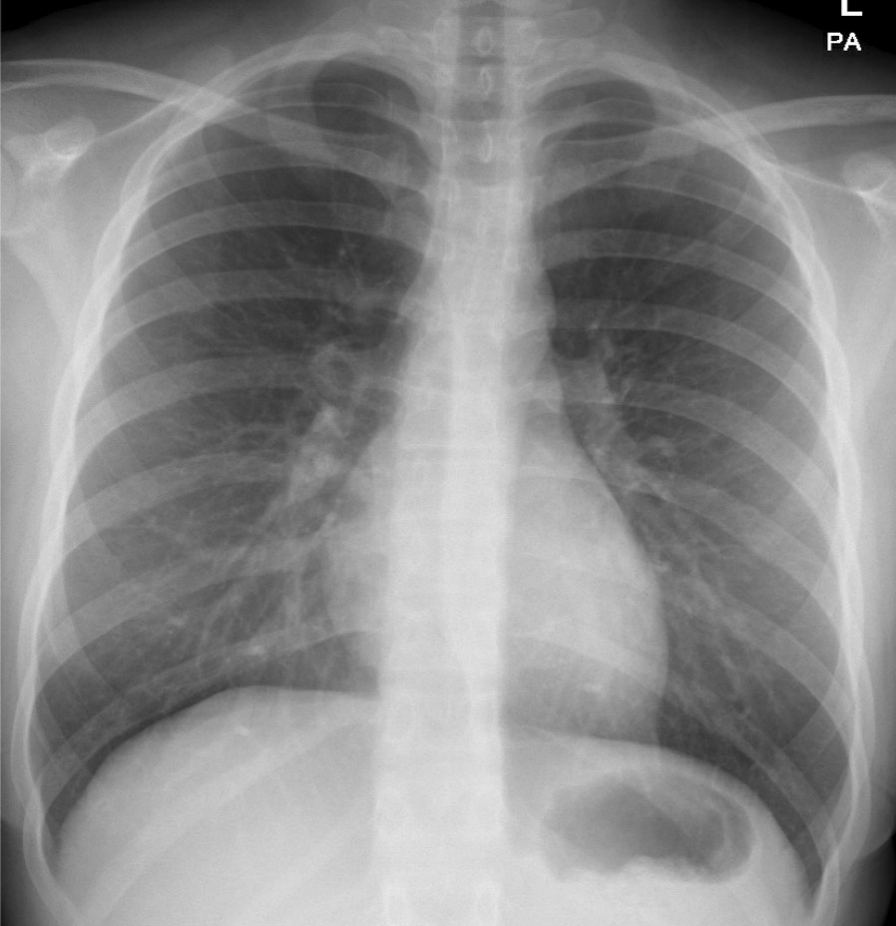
A 17-year-old girl with COVID-19 pneumonia based on positive RT-PCR test who presented with cough and rhinorrhea for 2 days. Frontal chest radiograph shows no radiographic abnormality. The patient’s serum biochemical parameters show a mildly elevated LDH level of 151 U/L
